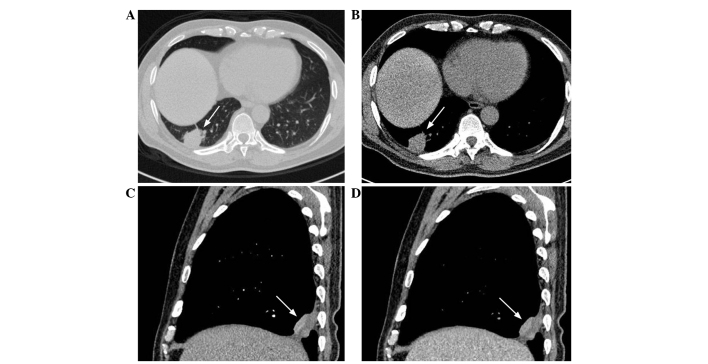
Plain chest computed tomography (CT) of a 54-year-old male with a pulmonary inflammatory myofibroblastic tumor. (A) Lung window setting and (B) mediastinum window setting revealing a well-defined, sub-pleural mass in the peripheral parenchyma of the right lower lobe. Contrast-enhanced CT images of the sagittal section in (C) the arterial phase and (D) the venous phase revealing high contrast enhancement in the solid portion of the mass, associated with the necrotic region.
